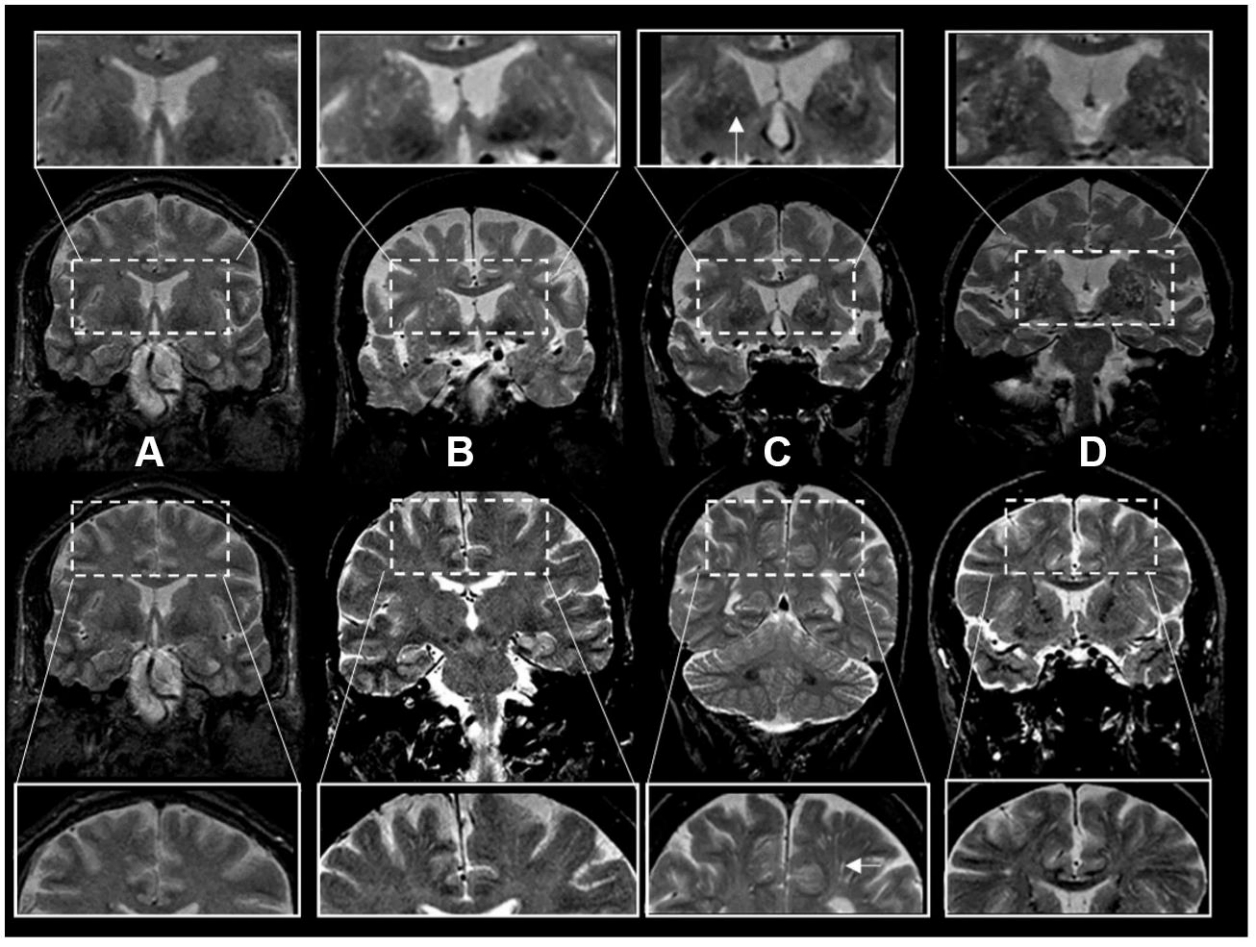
Enlarged perivascular space burden using ratings in coronal T2-weighted MRI sequences. *Top rows: basal ganglia region. Bottom rows: centrum semiovale region. The inserts represent a closer view. White arrows point to individual examples of enlarged perivascular spaces (panel C inserts). †Grades of ePVS burden are based on T2 weighted coronal views. (A) Grade I (0-10), (B) Grade II (10-20) (C) Grade III (20-40) (D) Grade IV (40+).
